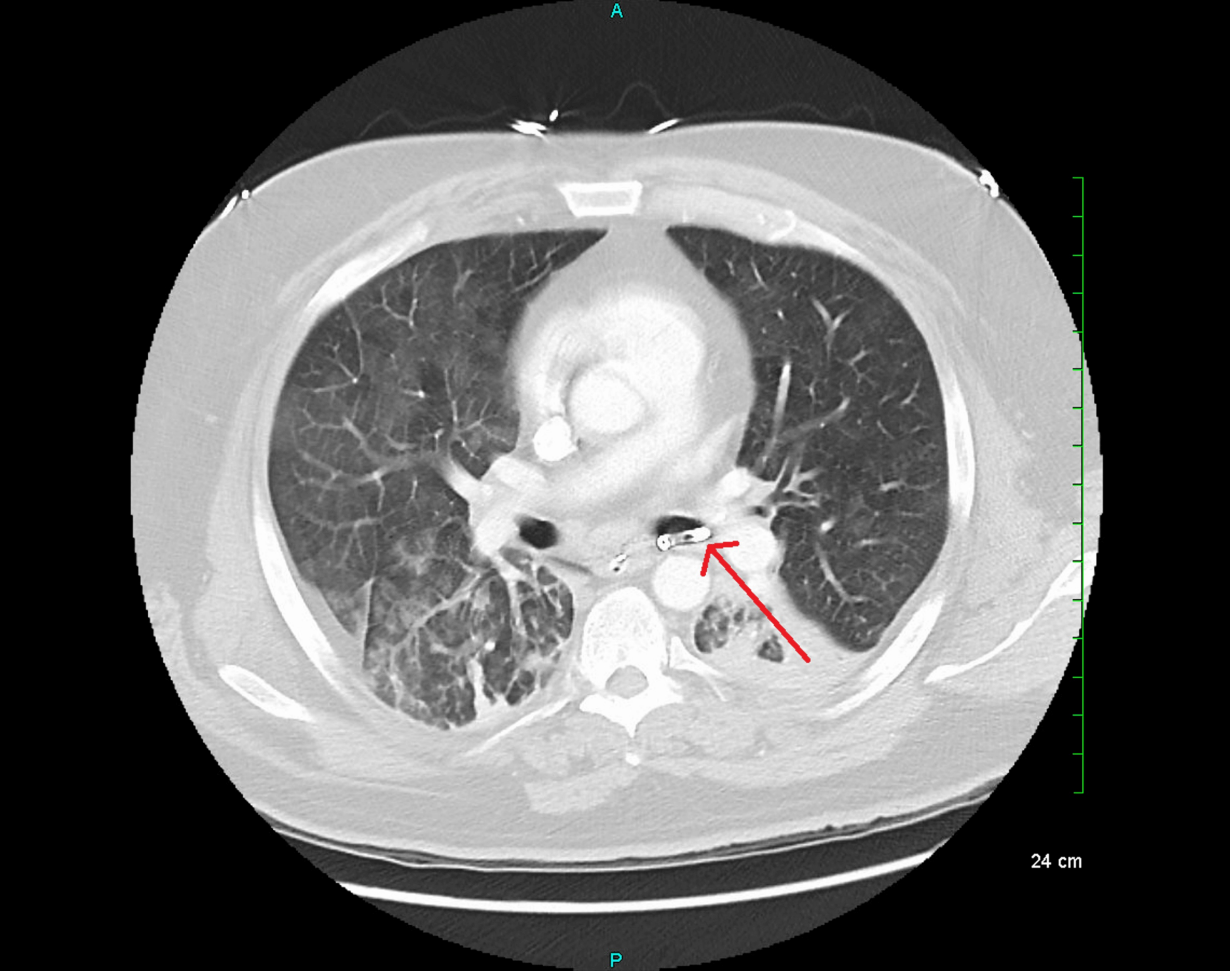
CT angiography chest (axial view) on day 7 with a pleural catheter in the left mainstem bronchus
